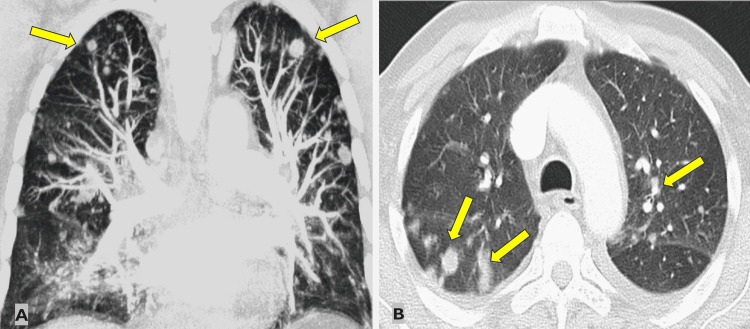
CT chest showing progressive pulmonary metastases (A and B) CT: computed tomography
